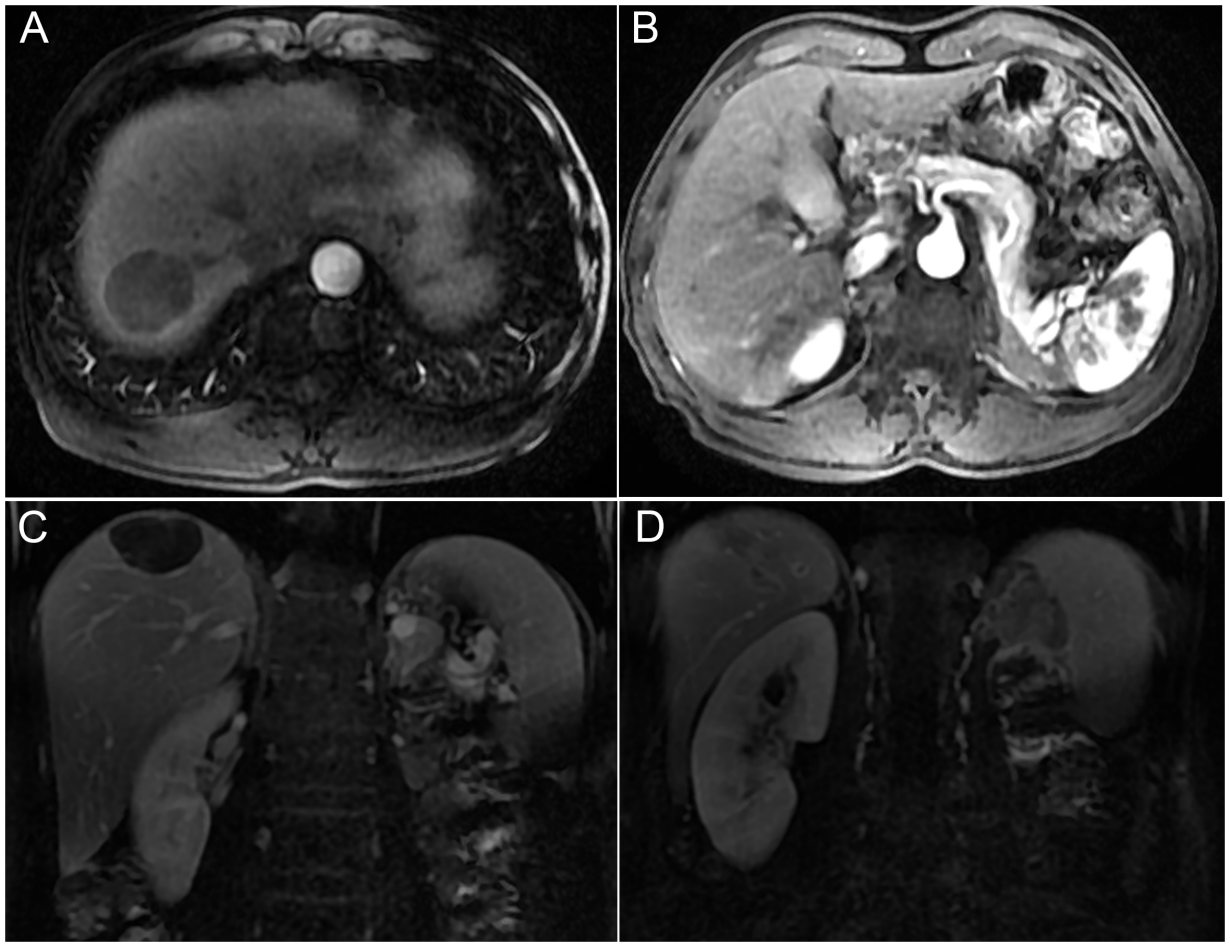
The enhanced MR images showed a complete response of the tumor after six months of follow-up. Axial and coronal MR images showed no tumor activity in both liver metastasis (A, C) and left retroperitoneal lymph node metastases (B, D).
